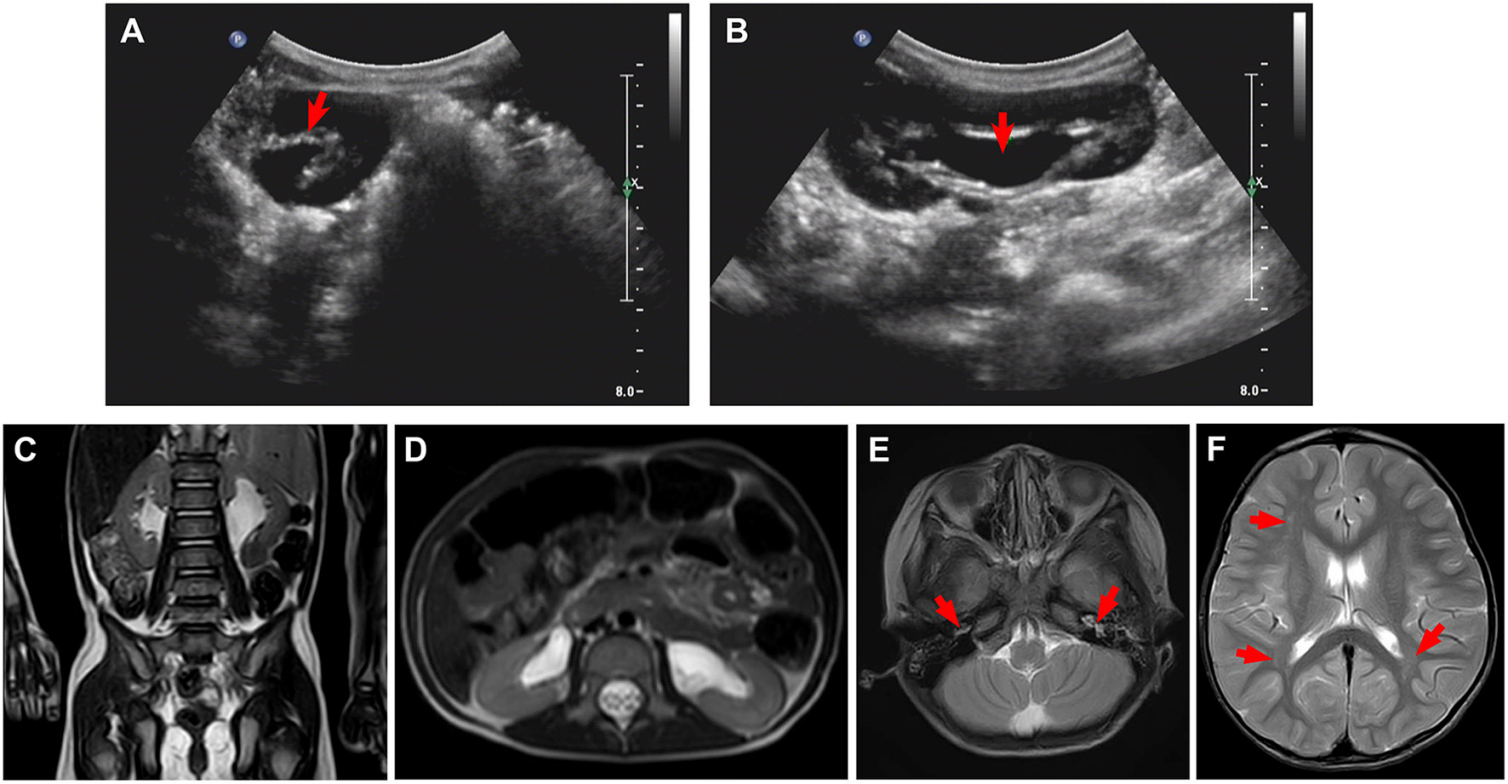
Ultrasound and magnetic resonance imaging (MRI) for kidneys and brain. (A,B) Ultrasound shows small crystals in the sinus of both kidneys, bilateral upper ureteral dilatation, and hydronephrosis. (C,D) MRI confirms bilateral hydronephrosis and ureteral dilatation, in particular, left side. (E,F) Cranial MRI scan shows bilateral mastoiditis (E, arrows) and abnormal patchy signal intensity in the anterior and posterior horns of the bilateral ventricles (F, arrows), indicating delayed myelination.
